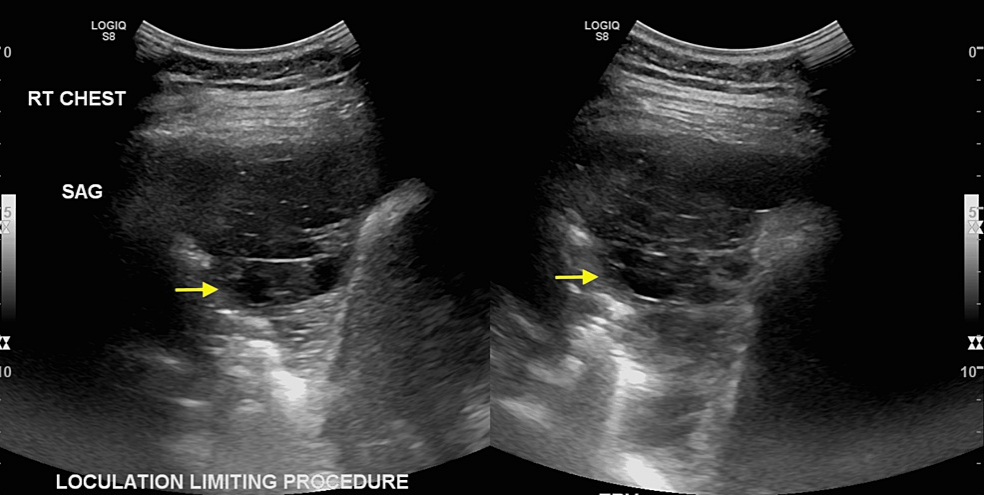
Ultrasound showing a multiloculated right-sided pleural effusion (yellow arrows).
